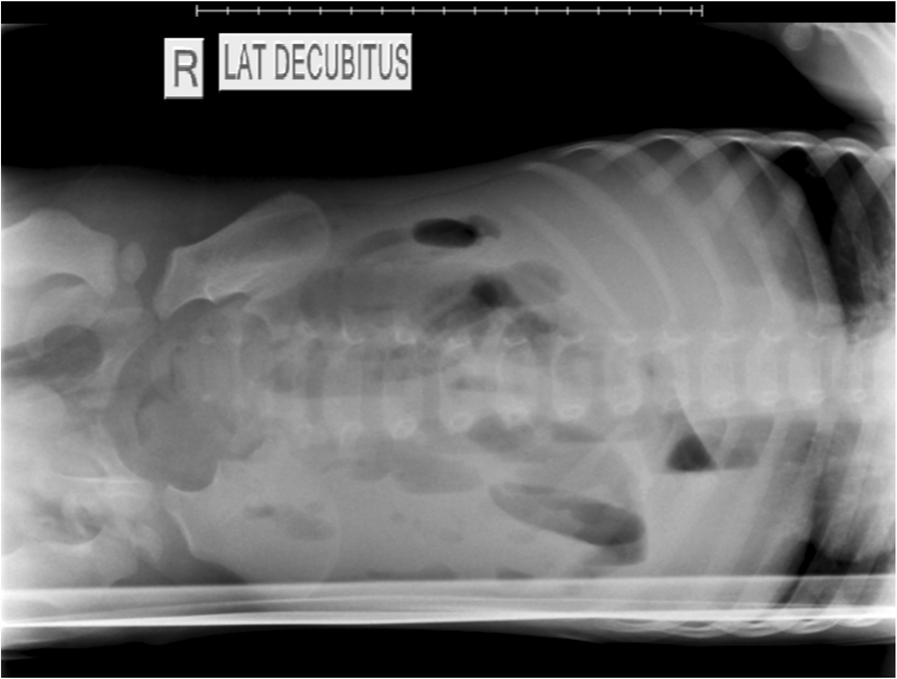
Lateral decubitus abdominal radiograph showing air-fluids levels with a sentinel loop of dilated small bowel
